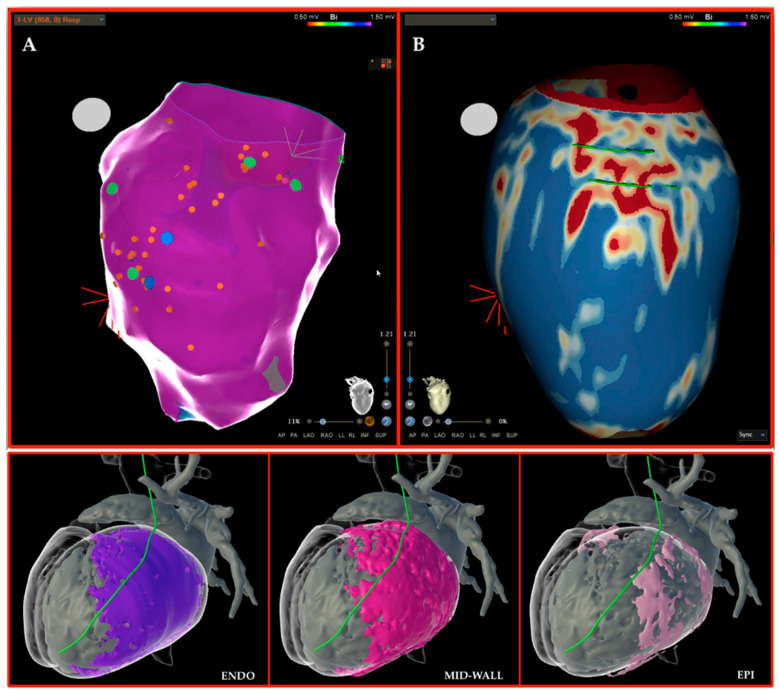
Examples of segmentation software applications. Upper Panel. (A) shows a case of an NICM patient and recurrent VT in which the endocardial substrate high-density mapping does not show low-voltage areas, except for a few abnormal ventricular EGM signals (green, blue and orange tags). Conversely, in (B), the ADAS 3D anatomical reconstruction of the LV epicardium obtained with processing LGE-CMR imaging is shown and, in particular, it is evident that scar localization and putative channels (green lines) are at the inferolateral basal wall. On the basis of this, the patient was submitted to an epicardial procedure. Lower Panel. A case of another patient with a genetically determined NICM and a transmural “ring-like” scar mostly affecting the lateral wall. From left to right, endocardial, mid-wall and epicardial layers are displayed, and it is clear that the major extension of the scar is in the endocardial/mid-wall layers rather than the epicardial one. In this patient, an inHeart reconstruction of the anatomical structures and the scar was made with processing CT-scan and late iodine enhancement imaging. The left phrenic nerve is shown in green.
